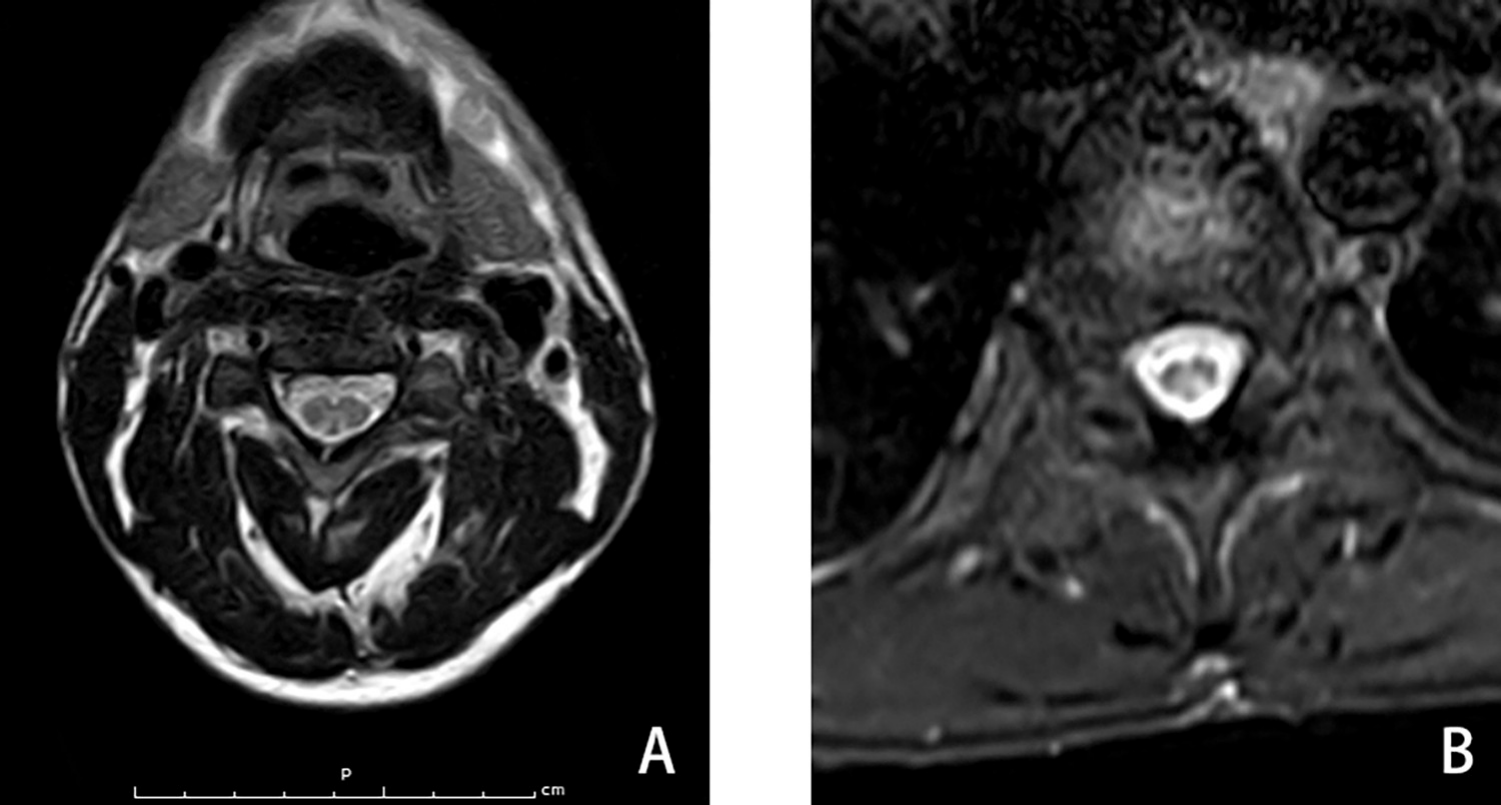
Spinal cord magnetic resonance imaging of case 18. Transverse sections of spinal cord showing “V” inverted T2 hyperintensity in the cervical spinal cord (A) and ellipse-shapes T2 hyperintensity in the thoracic spinal cord (B).
